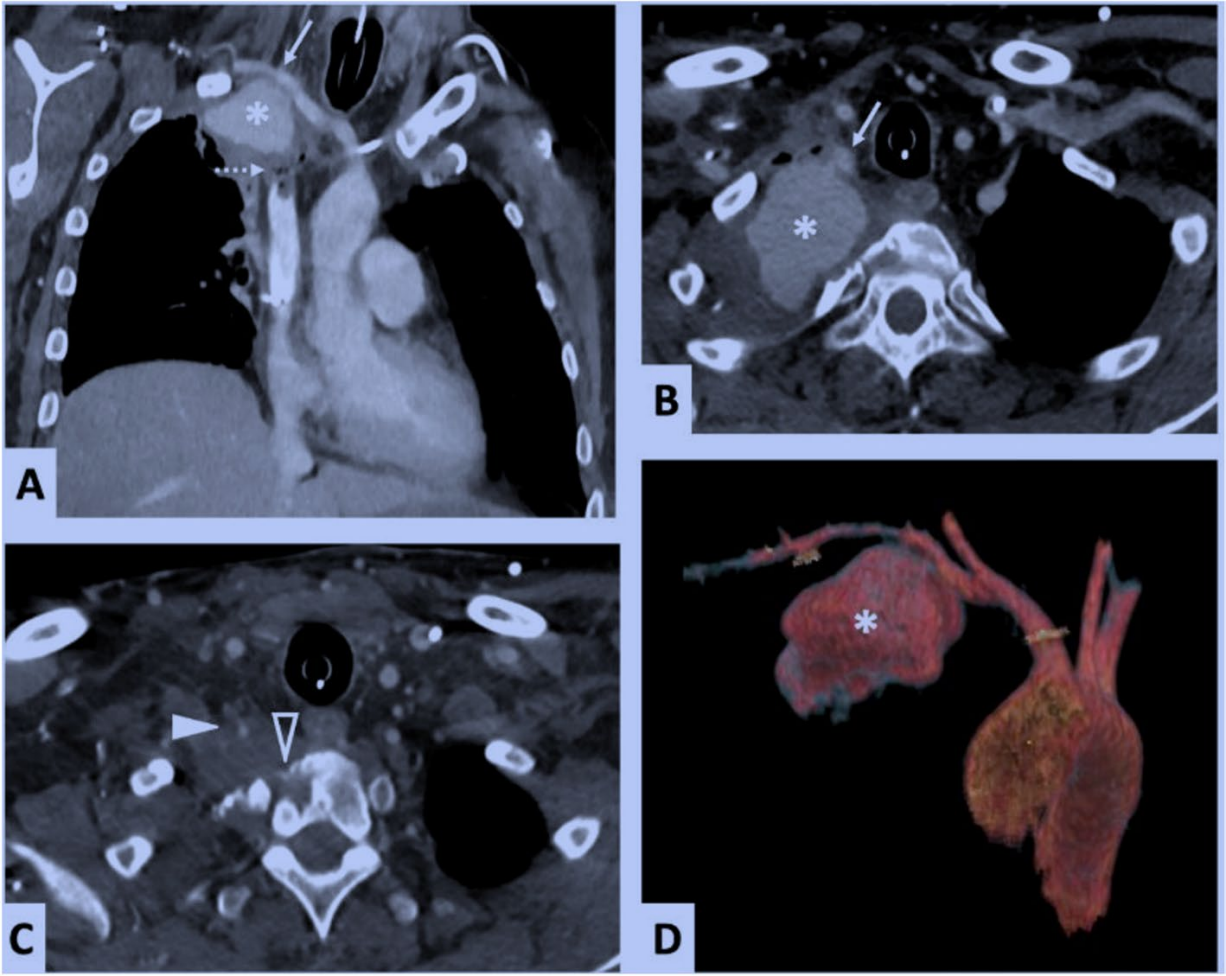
Thoracic CT angiography in the arterial phase after contrast agent injection, coronal slice (1-A), axial slices (1-B and 1-C), and volume rendering (1-D). Pseudoaneurysm of the right pulmonary apex (1-A, 1-B and 1-D, asterisk) contained by the edges of the Pancoast tumor, due to rupture of the posterior-inferior wall of the right SCA (1-A and 1-B, solid arrow). Tumor invasion of the mediastinum with thrombosis of the superior vena cava (1-A, dotted arrow), invasion of the right lateral part of the T1 vertebral body (1-C, hollow arrowhead), and encasement of the V1 segment of the right VA (1-C, solid arrowhead)
